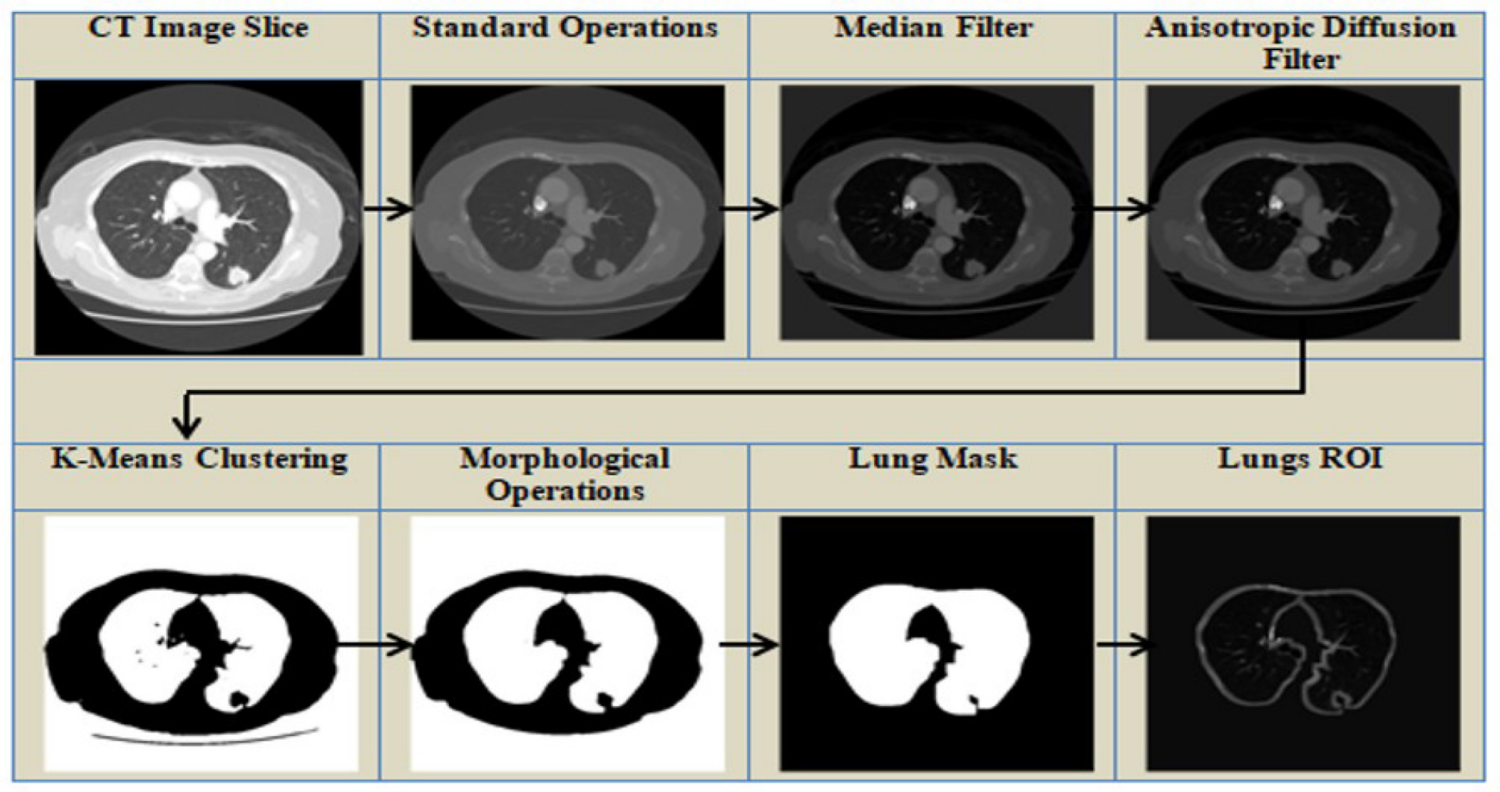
Pre-processing of the CT Scans to segment lungs.
